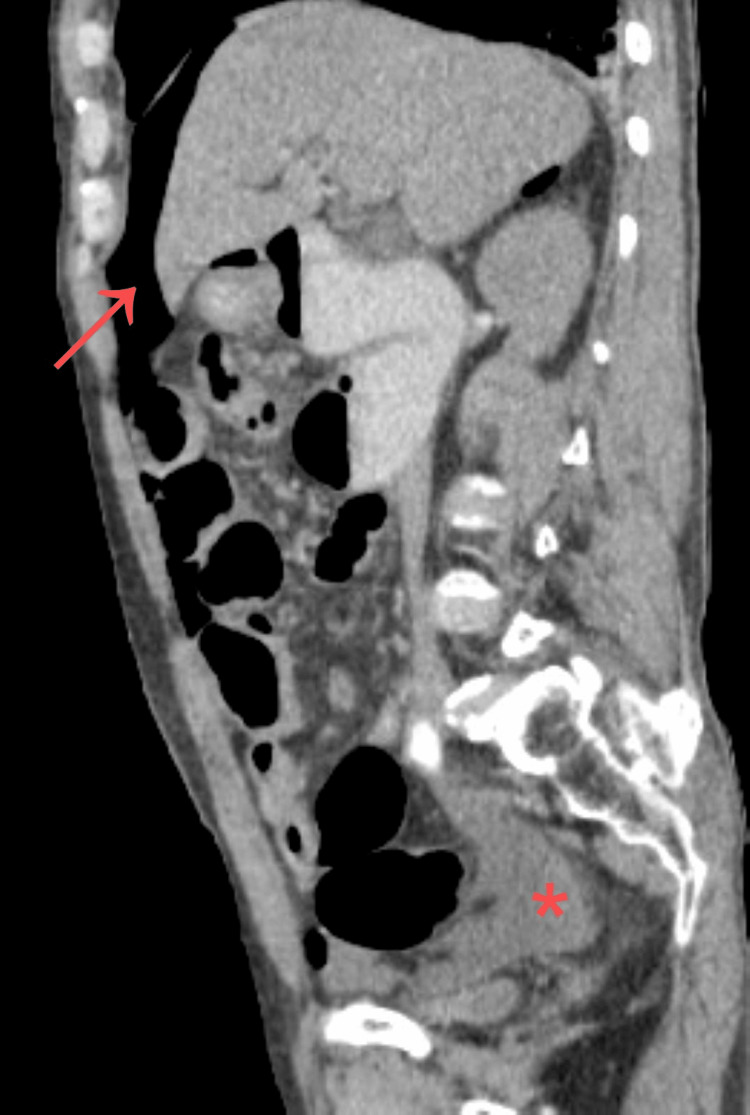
Pneumoperitoneum and pelvic fluid collection. Sagittal contrast-enhanced computed tomography image showing anterior pneumoperitoneum, indicated by the arrow, and an associated pelvic fluid collection, indicated by the asterisk. These findings support the diagnosis of perforated ileal diverticulitis with secondary peritonitis.
